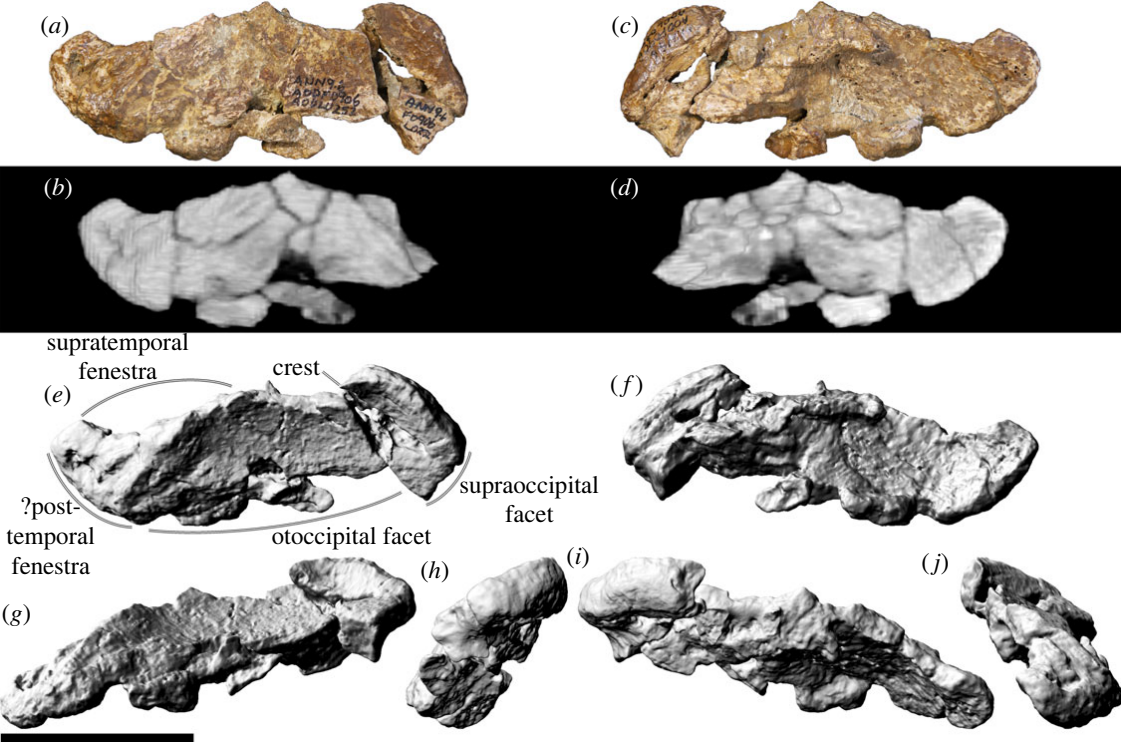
Diamantinasaurus matildae* referred left parietal (AODF 0906). (*a–d*) Left parietal in (*a*,*b*) posterior and (*c*,*d*) anterior views. (*a*) and (*c*) are photographs, (*b*) and (*d*) are three-dimensional models derived from CT data. (*e–j*) Three-dimensional models (derived from surface scans) of the left parietal in (*e*) posterior, (*f*) anterior, (*g*) ventral, (*h*) medial, (*i*) dorsal and (*j*) lateral views. Scale bar = 50 mm.
